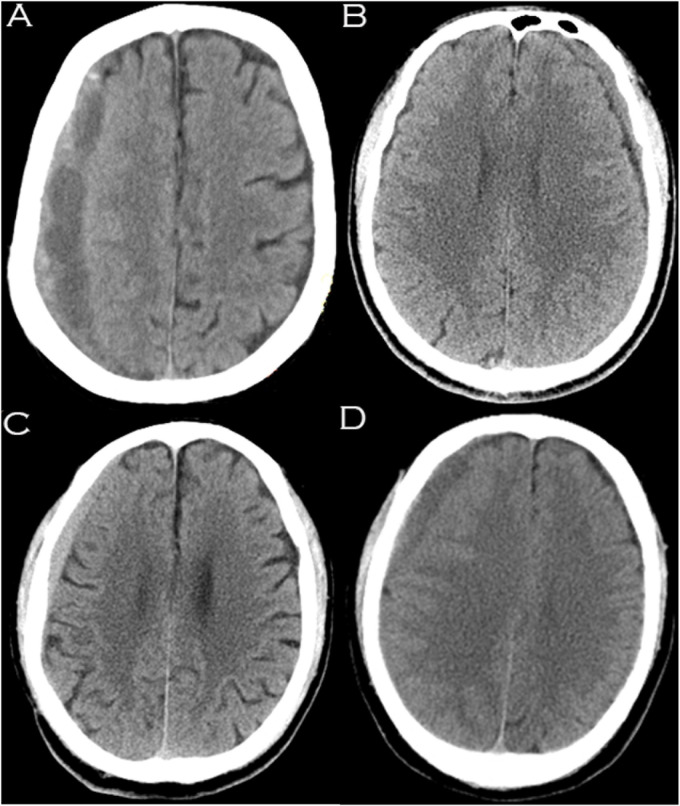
Hematomas of different densities as visualized on computed tomography images. (A) Mixed density hematoma; (B) Isodensity hematoma; (C) Hyperdensity hematoma; (D) Low-density hematoma.
